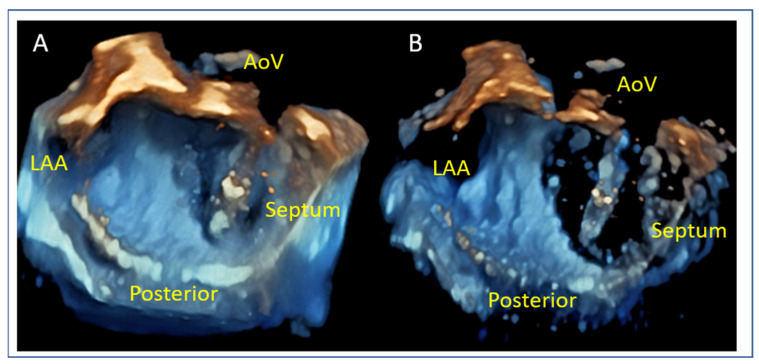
Three-dimensional mitral valve shows device orientation. (A) Perpendicularity control after crossing mitral valve to check optimal orientation. (B) After the device is advanced into the left ventricle (LV), its orientation can be assessed using 3D echocardiography. Reducing the gain during imaging enhances the clarity of the clip and its position, allowing for more precise visualization of its alignment in relation to the coaptation line. AoV: aortic valve, LAA: left atrial appendage.
